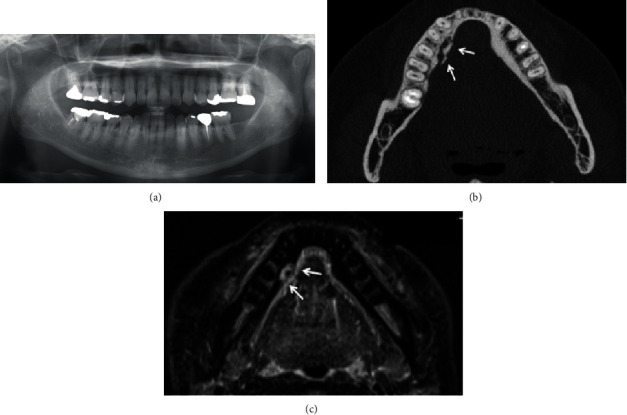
Imaging findings. (a) Panoramic radiography showed no obvious bone destruction or marginal and apical periodontitis. (b) Axial computed tomography depicted a sequestrum measuring approximately 25 mm (arrow). (c) Axial magnetic resonance short T1 inversion recovery imaging showed abnormal intramedullary signal intensity (arrow) on the lingual side of the right lower premolar.
